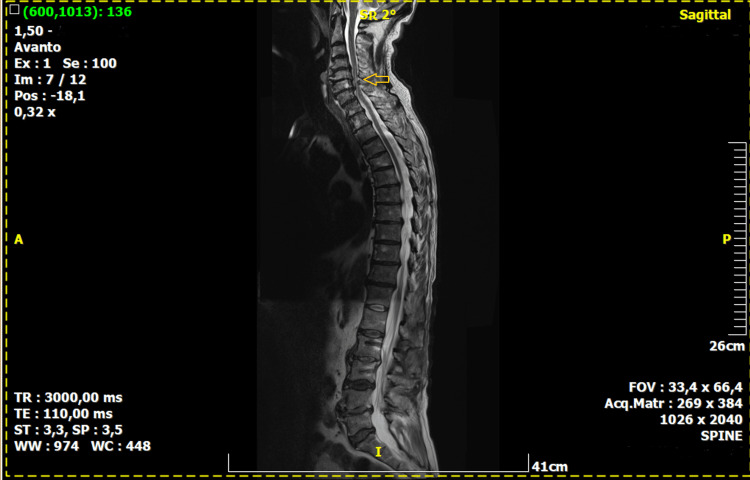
Full-body MRI scan Full-body MRI scan of the patient with the presence of asymptomatic cervical myelopathy
